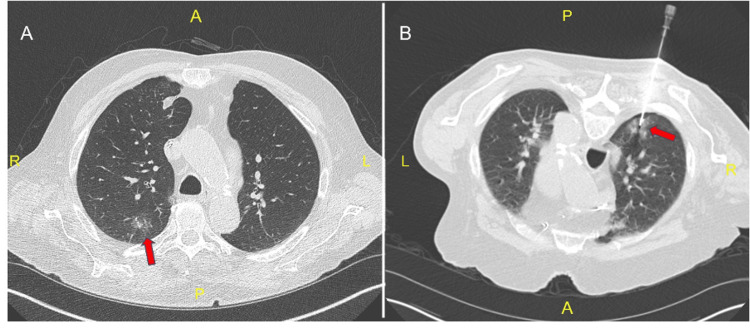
A) Chest computed tomography axial view showing a 20 x 14 mm sub-solid nodule with solid components measuring 4 mm in the right upper lobe. B) Computed tomography image obtained during a CT-guided biopsy, showing a biopsy needle targeting a subsolid nodule in the right upper lobe.
